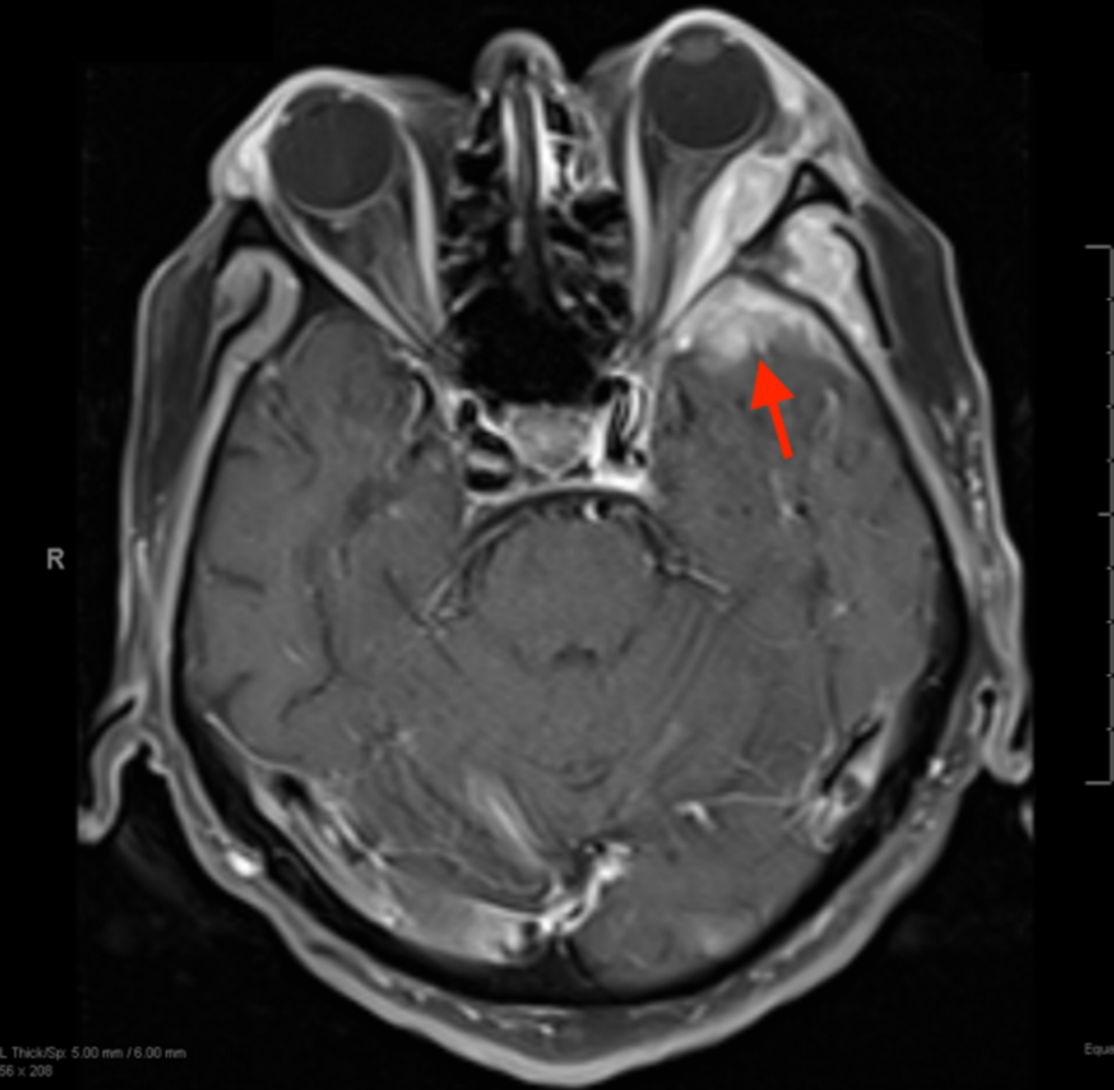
Axial T1 MRI of the brain with contrast revealing a 4.3 cm enhancing mass (red arrow) in the left anterior temporal and lateral orbital wall. MRI: Magnetic resonance imaging
